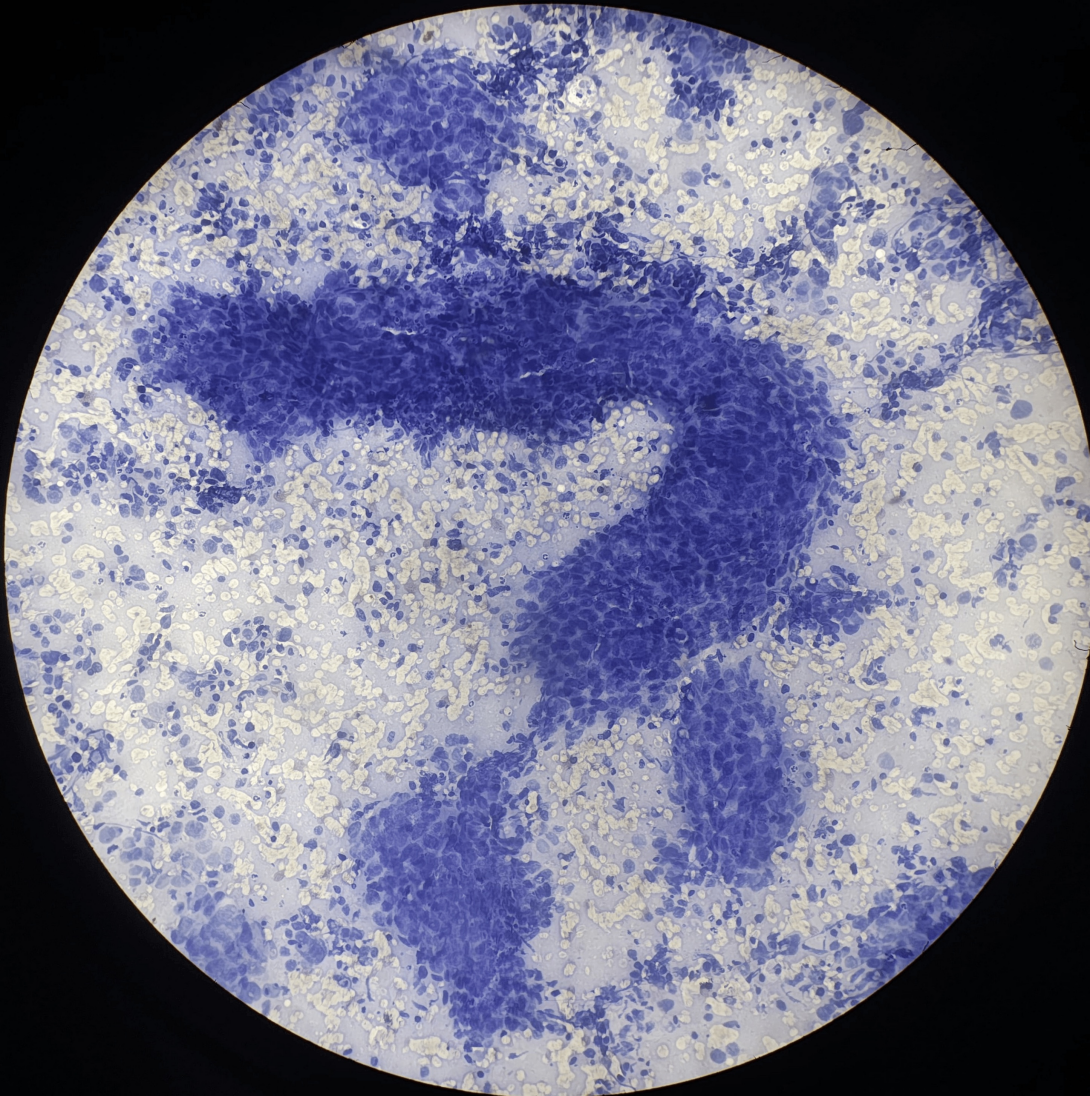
USG-guided FNAC showing thick trabeculae of cells in HCC USG: Ultrasonography; FNAC: Fine-needle aspiration cytology; HCC: Hepatocellular carcinoma
